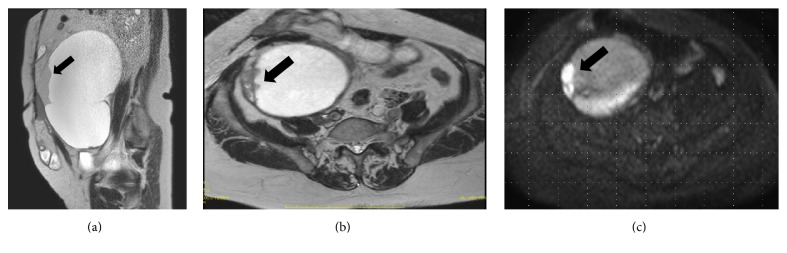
Pelvic plain magnetic resonance imaging. (a) T2-weighted image in the sagittal plane. A cyst measuring 21 × 19 × 12 cm is observed. It is partially solid. (b) T2-weighted image in the horizontal plane. (c) Diffusion-weighted image in the horizontal plane. The solid components observed on the T2-weighted image show high signal intensity on the diffusion-weighted image (*⬅*). The cyst is suspected to be a malignant lesion.
